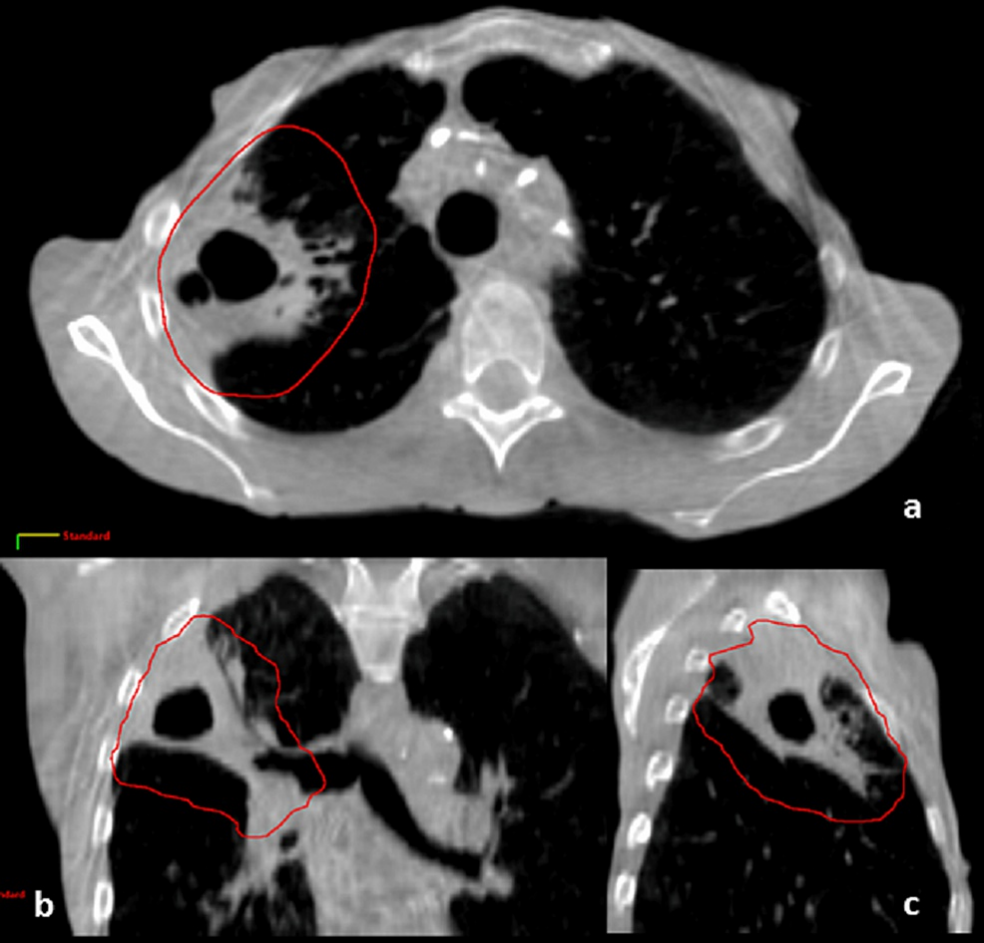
Final treatment CBCT images showcasing a considerable decrease in the size of the tumor. a, b, and c: axial, coronal, and sagittal planes (CBCT at fraction 30), outlined structure in red: original GTV at fraction 1 CBCT: cone beam computed tomography, GTV: gross tumor volume
